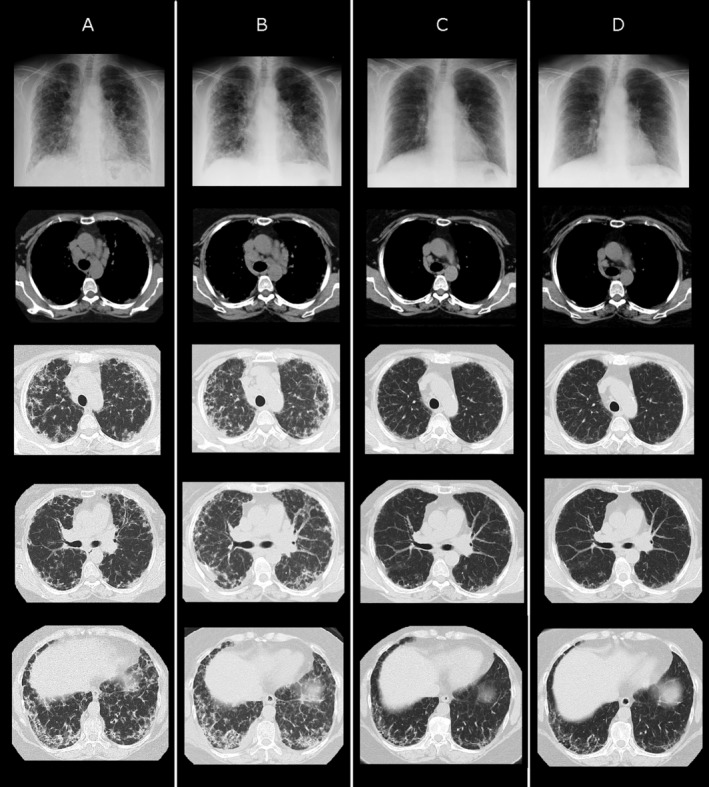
Time course of chest X‐ray and computed tomography. (A) On referral in April 2018. (B) At the initiation of treatment in the middle of August 2018. (C) Four months after the initiation of treatment (middle of December 2018). (D) One year after the initiation of treatment (August 2019).
